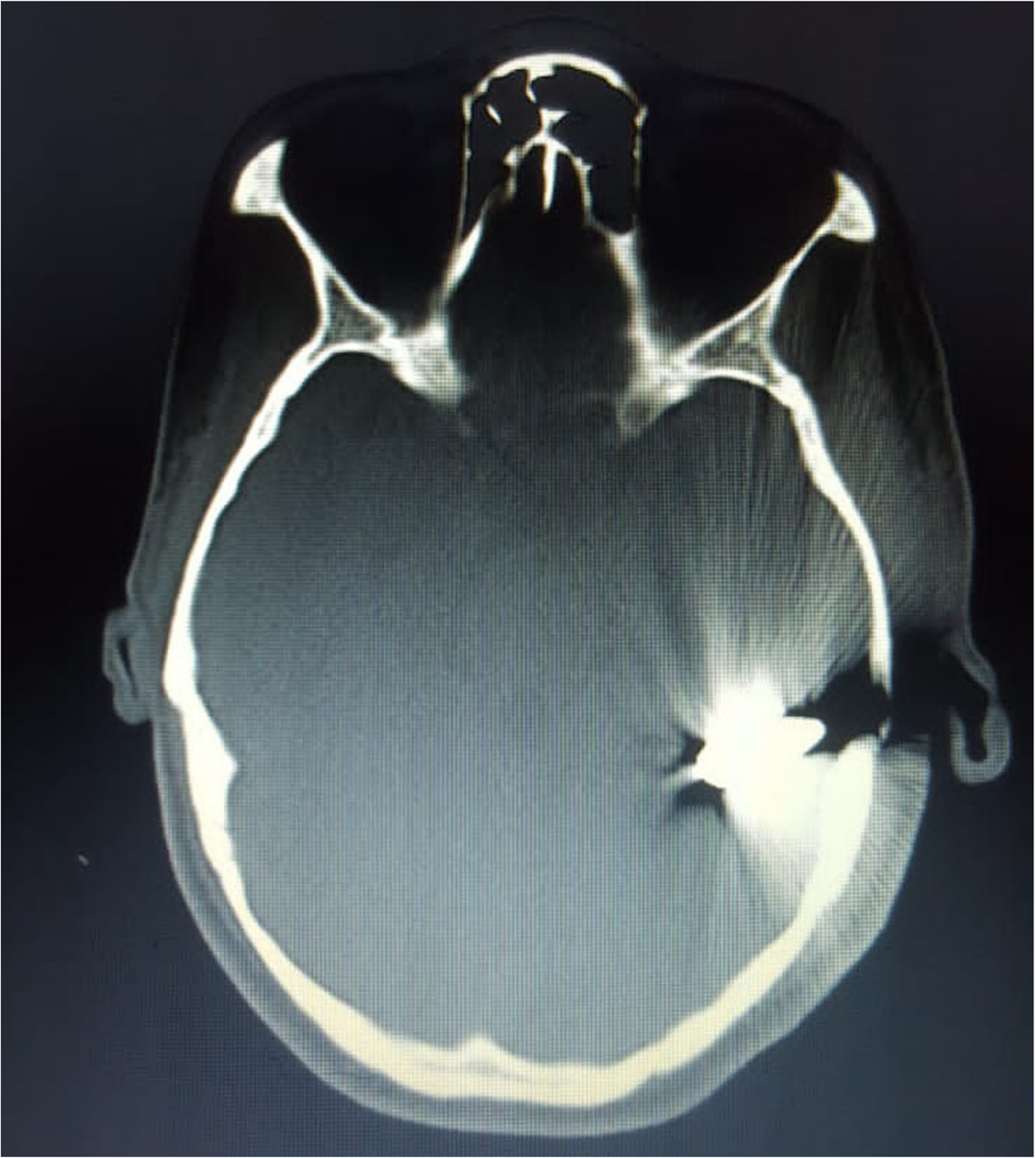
Bone window CT of a patient with PCCI showing a retained bullet in the cranium
